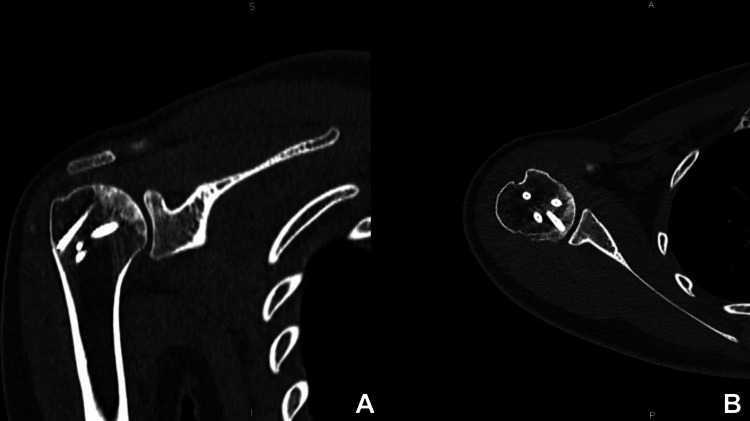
Follow up CT: coronal (A) and axial (B) slides The CT shows adequate bone consolidation at the one-year follow-up visit.
